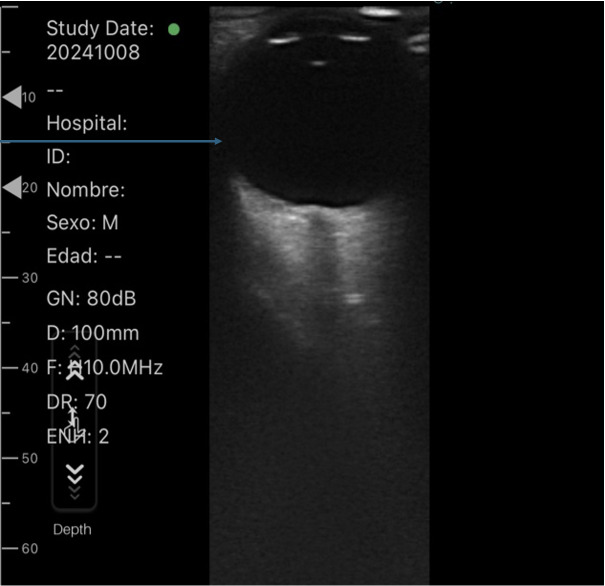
Ocular point of care ultrasound (POCUS) of the left eye. The blue arrow indicates a healthy left globe.
